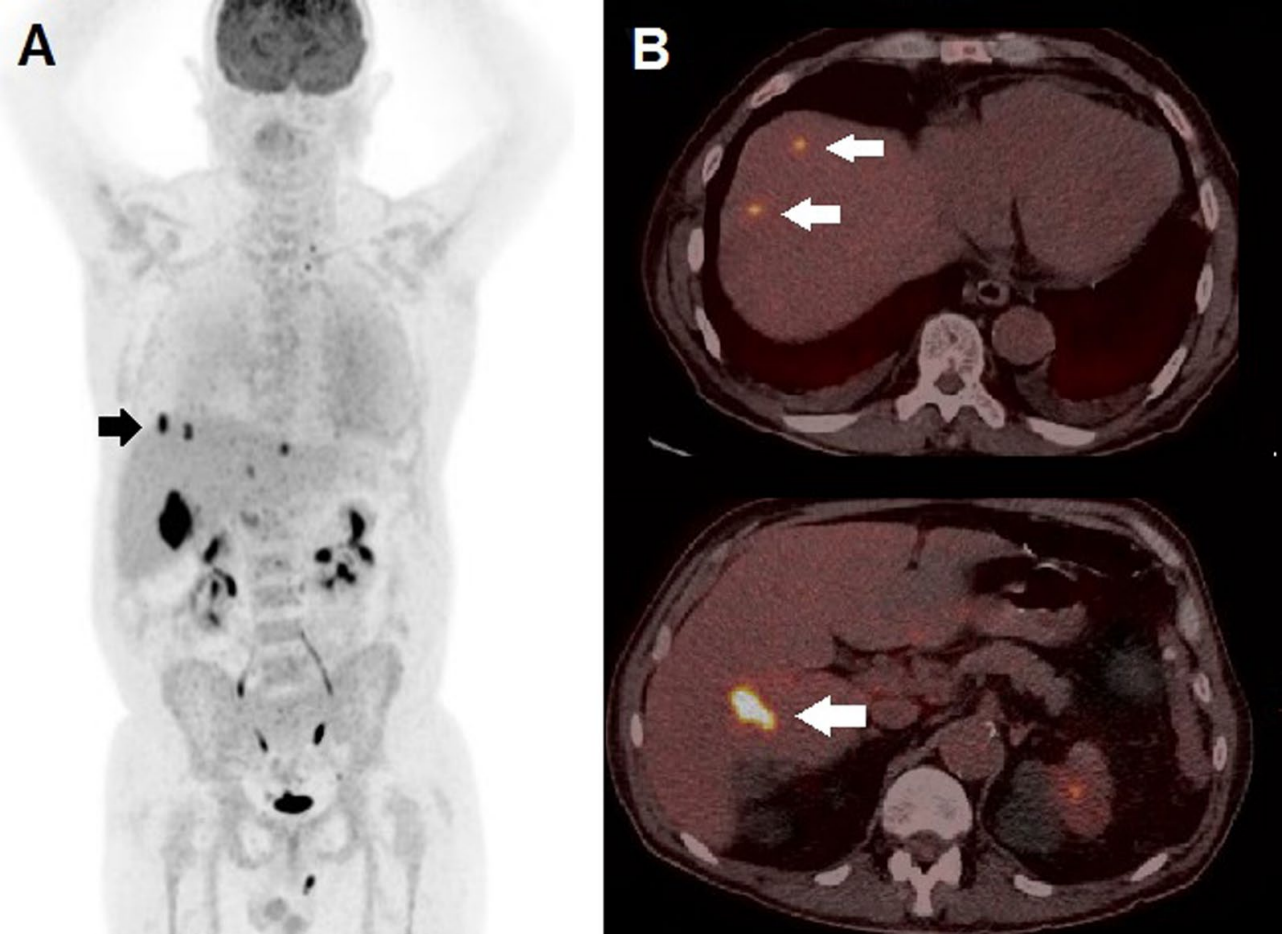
^18^F-FDG PET/CT Whole Body (a) performed after splenectomy demonstrated focuses of tracer uptake in the liver, as then detailed by fused PET/CT axial slices (b) that demonstrated hypermetabolic lesions (white arrows) in the hepatic dome (upper row) and in the 6th hepatic segment (lower row), with SUVmax of 25.3
